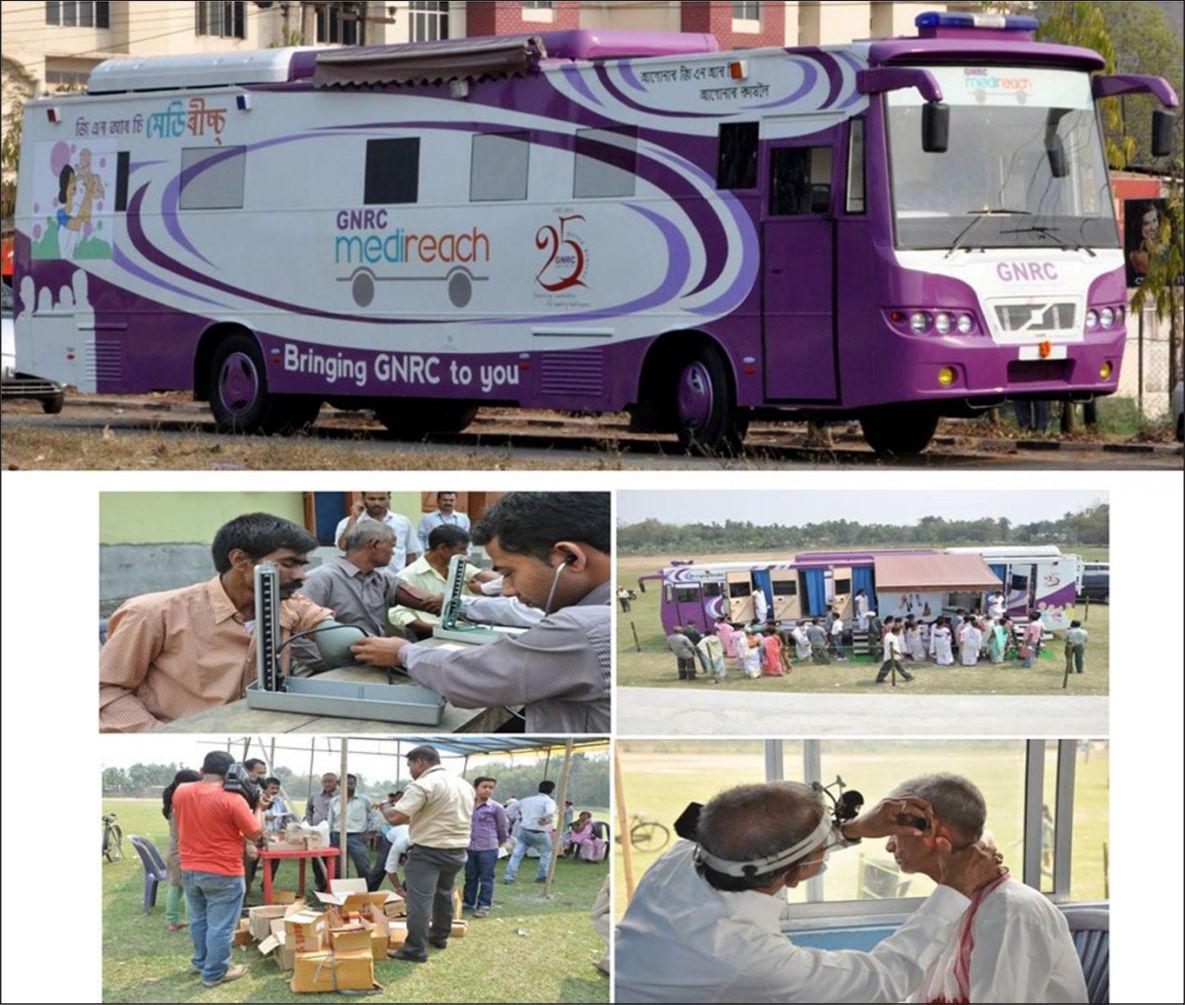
GNRC’s Medireach bus with ECGs, blood sugar, ultrasonography, X-ray, and pap-smear facility.
